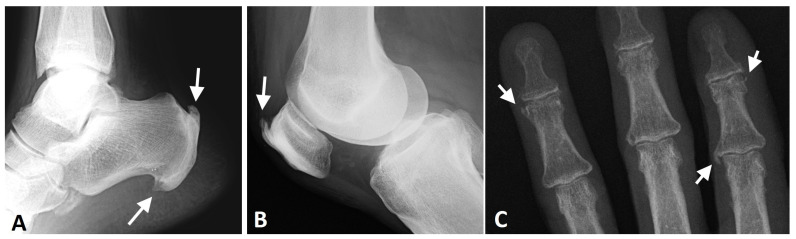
Radiographs of the ankle, knee, and hand of a patient with thoracic DISH (not shown). (A) Lateral radiograph of the ankle, showing coarse, thick enthesophytes in the attachment of the Achilles tendon and plantar fascia to the calcaneus (arrows). (B) Lateral knee radiograph showing thick, coarse enthesophytes (arrow) in the attachment of the quadriceps tendon to the patella. (C) AP radiograph of the fingers, showing enthesophytes in the medial side of the 2nd metacarpophalangeal joint and 4th distal interphalangeal joint and the lateral side of the 2nd distal interphalangeal joint (arrows).
